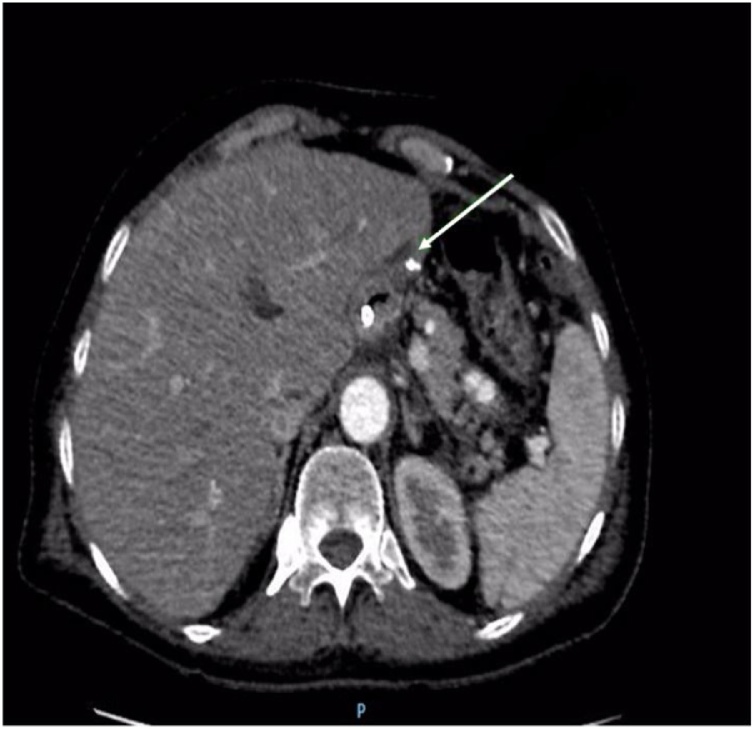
Abdomen CT for staging of gallbladder adenocarcinoma: Wall thickening in the proximal gastric segment with exophytic component close to the lesser curvature, measuring 5 × 4 cm (arrow).
